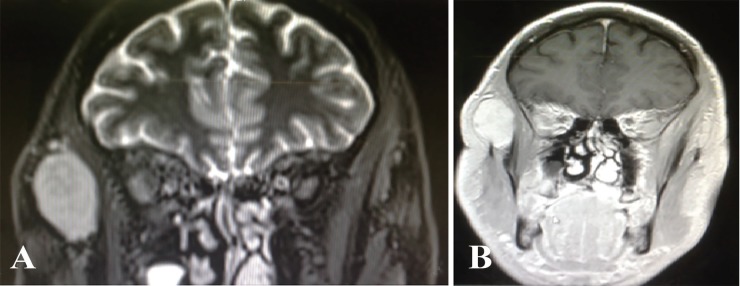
MRI shows a large soft tissue mass within the right temporalis muscle with extension to right infratemporal fossa. (A): The tumor has isointense signal on T1 weighted sequences. (B): The tumor has a high signal on T2 weighted sequences
